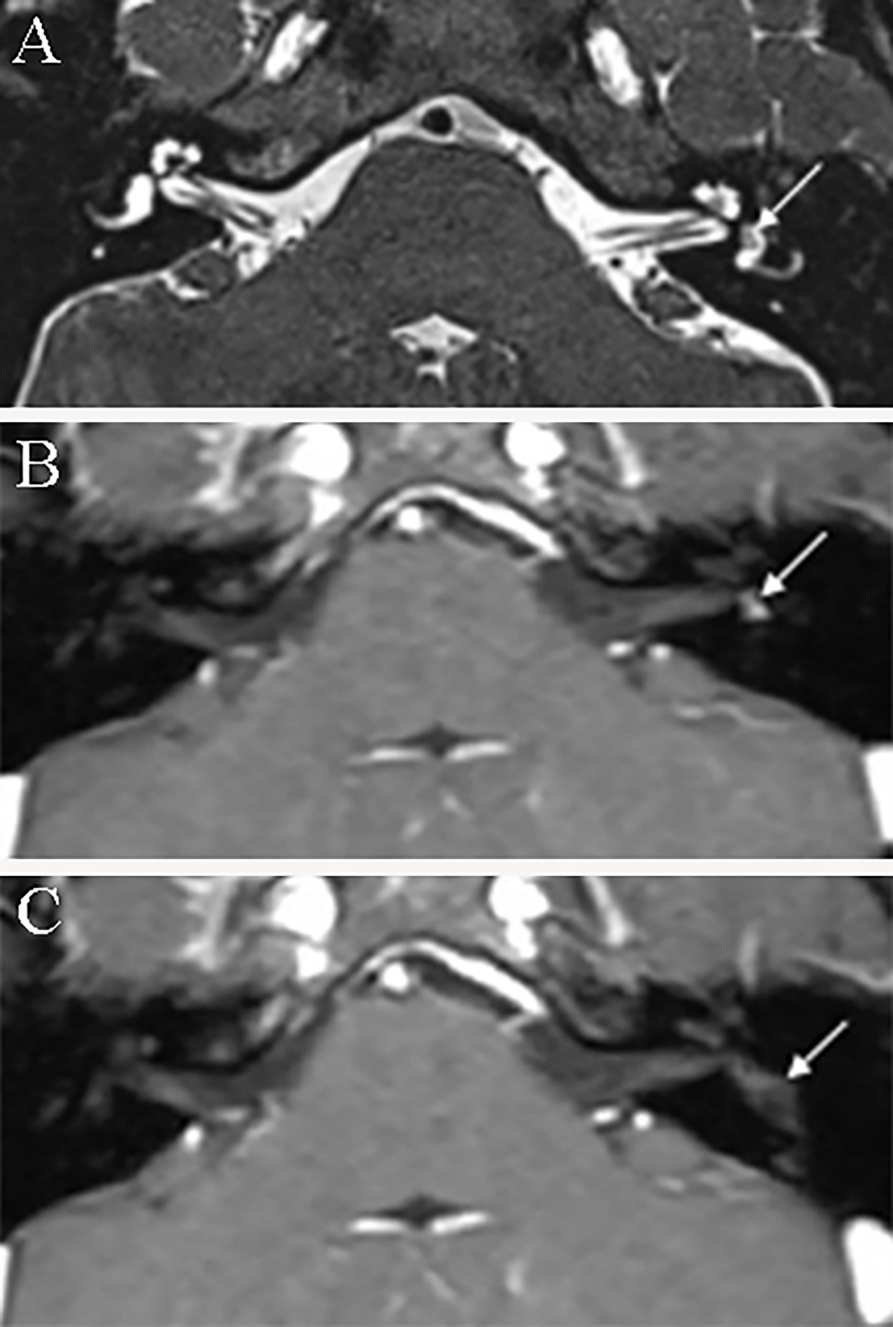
Axial magnetic resonance imaging of patient 4 before and after surgery. (A) T2-3D-space MRI reveals a hypointense filling defect within the left vestibule (arrow). (B) Enhanced T1-weighted MRI demonstrates a mass (arrow) within the vestibular cavity before surgery. (C) Enhanced T1-weighted MRI reveals no enhanced mass within the vestibule (arrowhead) of the tumor at 6 months postoperatively.
